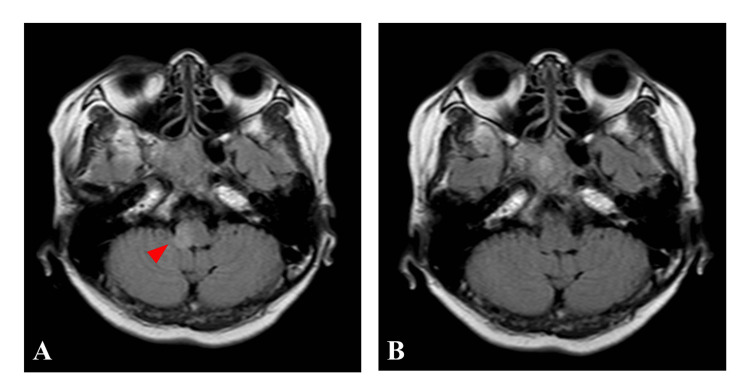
Post-operative images of Case 1 A: Fluid-attenuated inversion recovery sequence (FLAIR) images of MRI obtained one hour after the procedure show a high-intensity lesion in the right lateral medulla oblongata. B: A follow-up FLAIR image of MRI obtained 17 days after the procedure shows no high-intensity lesion in the right lateral medulla oblongata.
